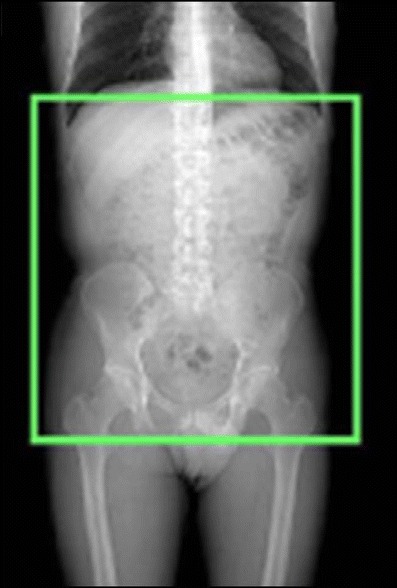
Scanning coverage of unenhanced and enhanced CT-acquisition
